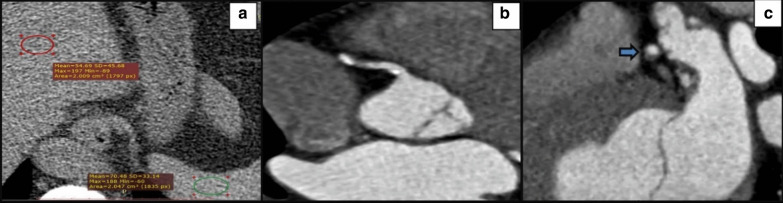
A 65-year-old lady with diabetes shows: a Axial non-enhanced CT image demonstrates attenuation measurements of the liver and spleen shows a diffuse fat accumulation in the liver, with a mean liver attenuation of 54.6 HU and a mean spleen attenuation of 70.4 HU. b Coronary CT angiography images shows a plaque with spotty calcium and c Coronary CT angiography shows a non-calcified plaque demonstrates a napkin-ring sign(arrow) with a central low-attenuation area, surrounded by a peripheral rim of higher attenuation
